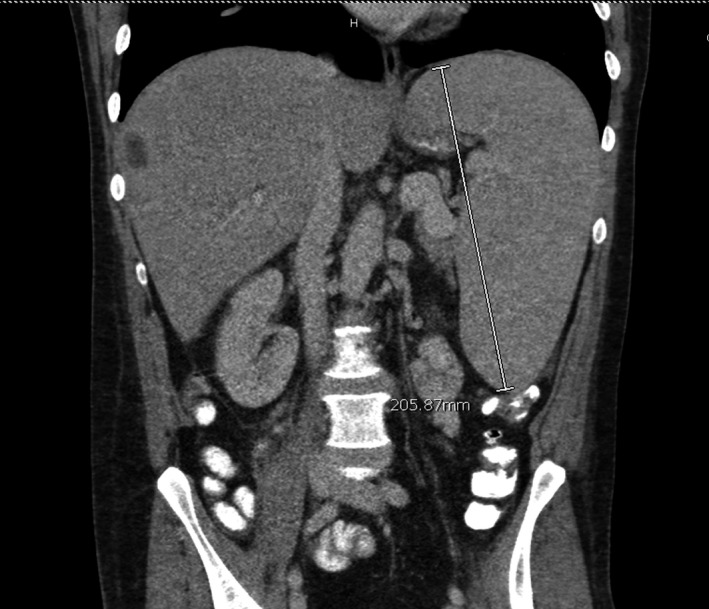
CT scan of the abdomen demonstrates hepatomegaly and splenomegaly
